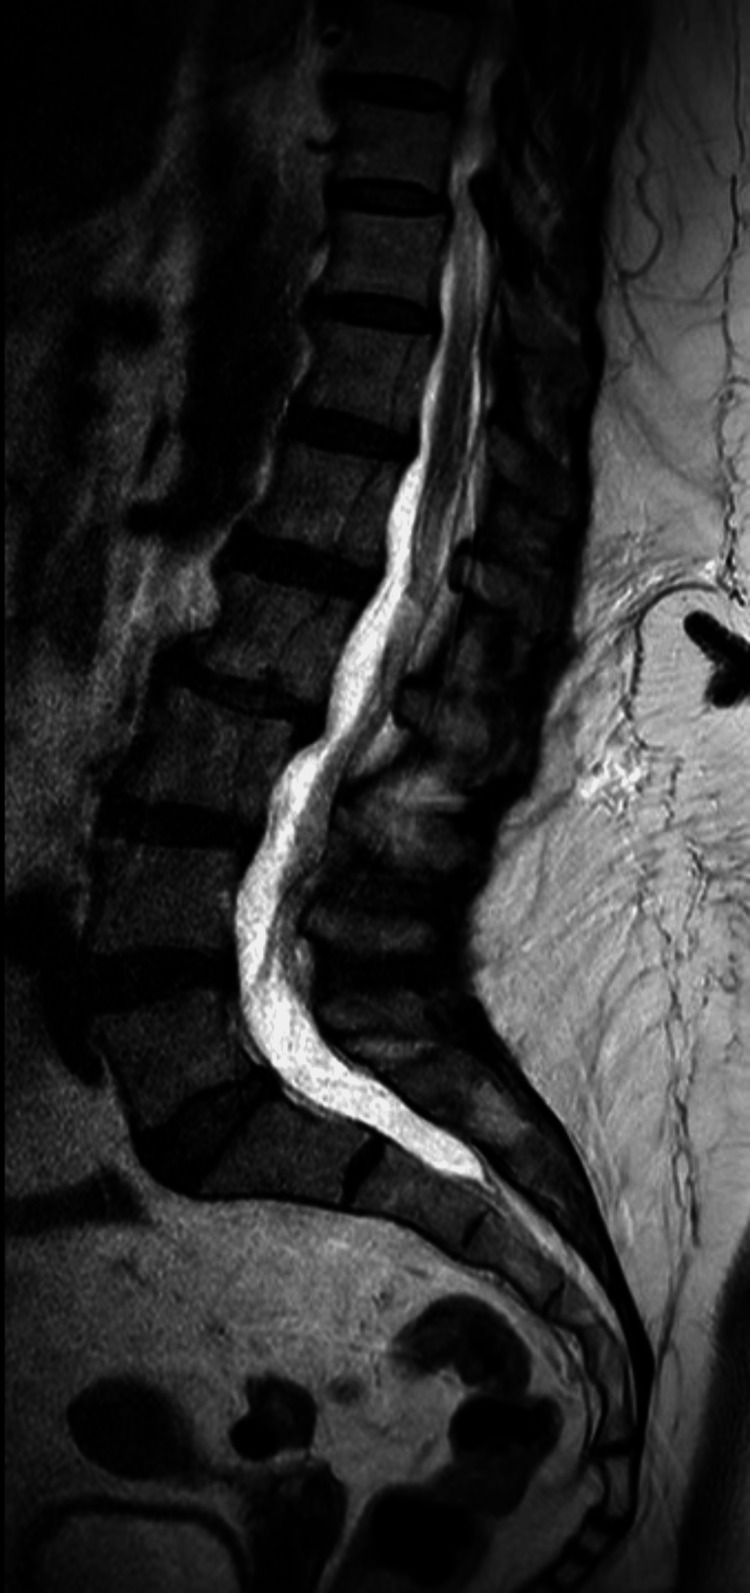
MRI of the lumbosacral spine showing no evidence of myelitis MRI of the lumbosacral spine was also done to rule out myelitis as the patient was complaining of lower back pain.
